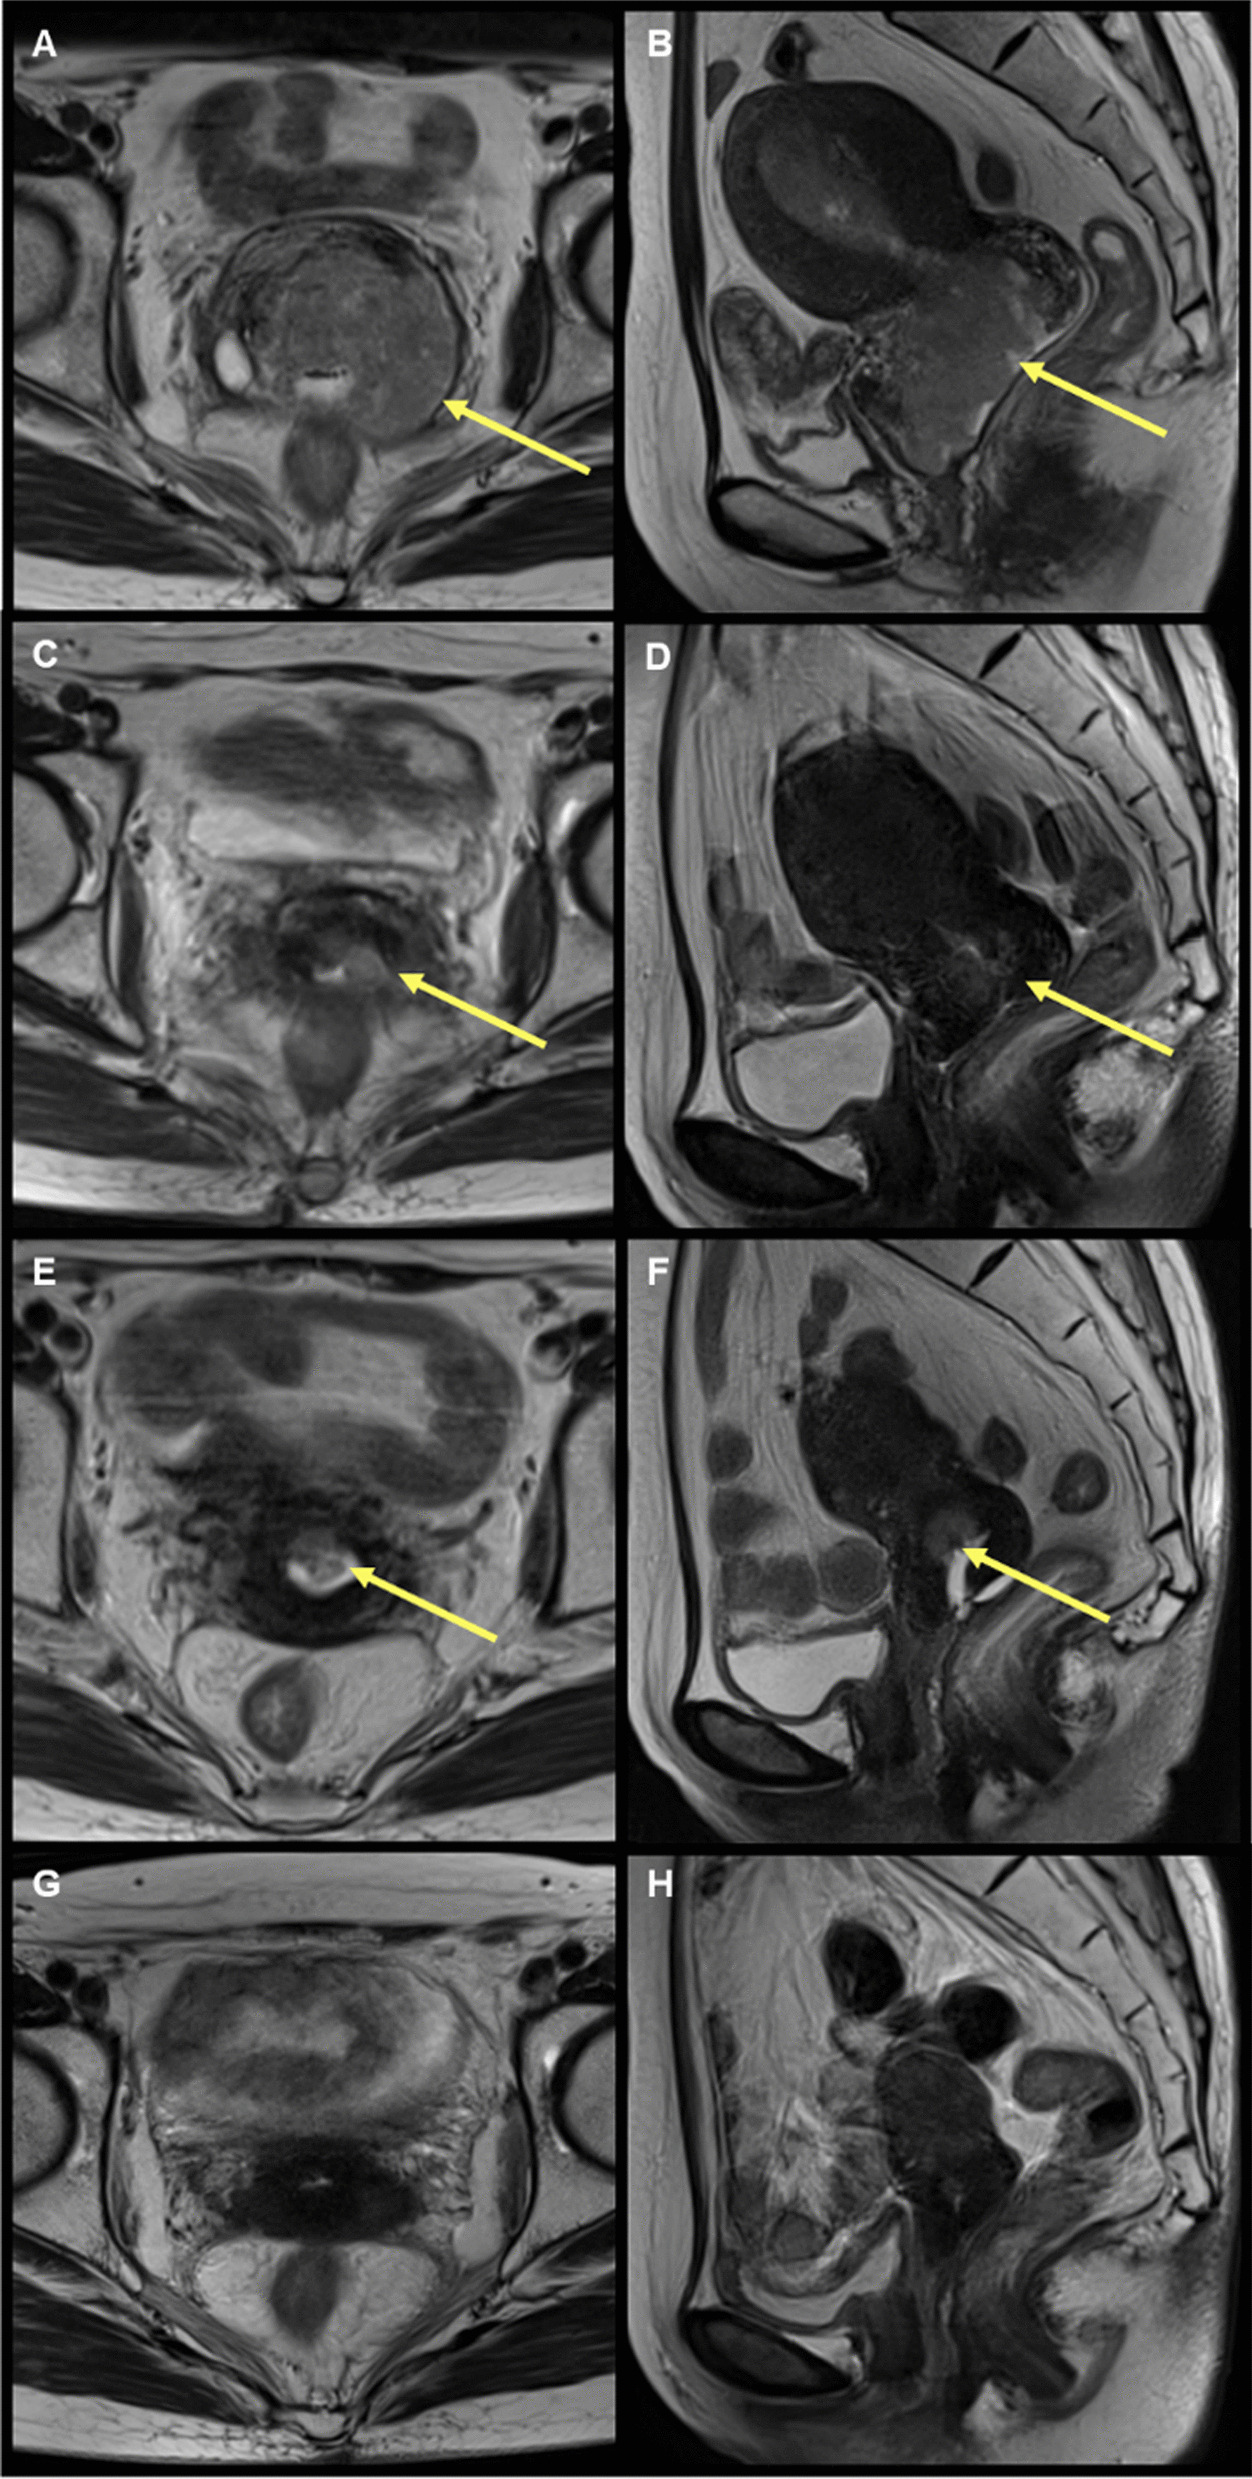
T2-weighted magnetic resonance images of the primary tumor (yellow arrows). Time of diagnosis: axial (A) and sagittal (B) planes. At 3 months: axial (C) and sagittal (D) planes. At 6 months: axial (E) and sagittal (F) planes. At 30 months: axial (G) and sagittal (H) planes
